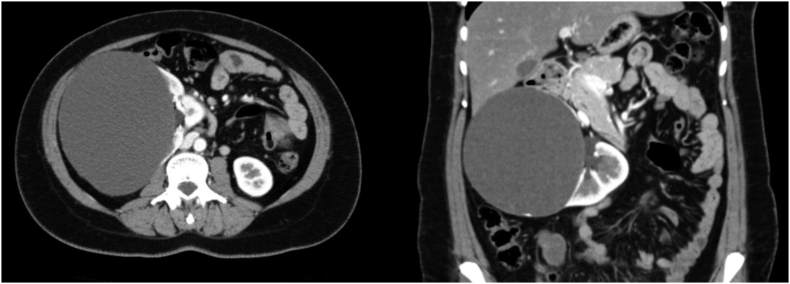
Contrast-enhanced CT showed a right simple renal cyst measuring 140 mm in diameter.
